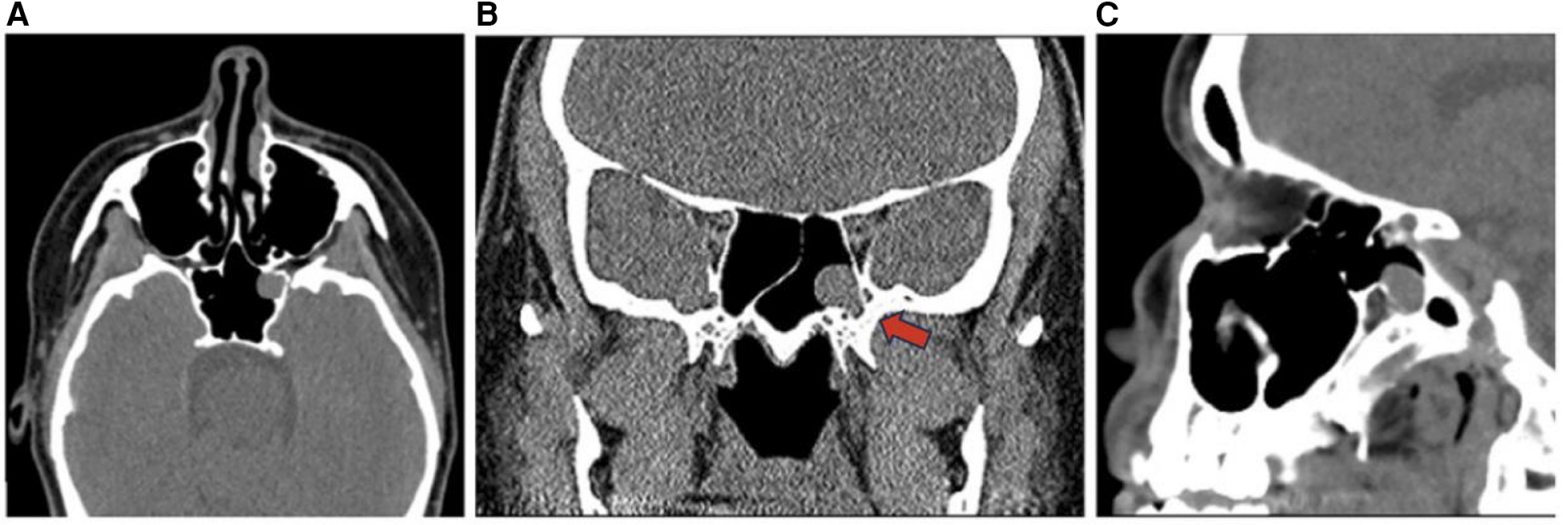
Mucous retention cyst axial (A), sagittal (B), and coronal (C) CT head images demonstrate a retention cyst in the left sphenoid sinus (red arrow).
